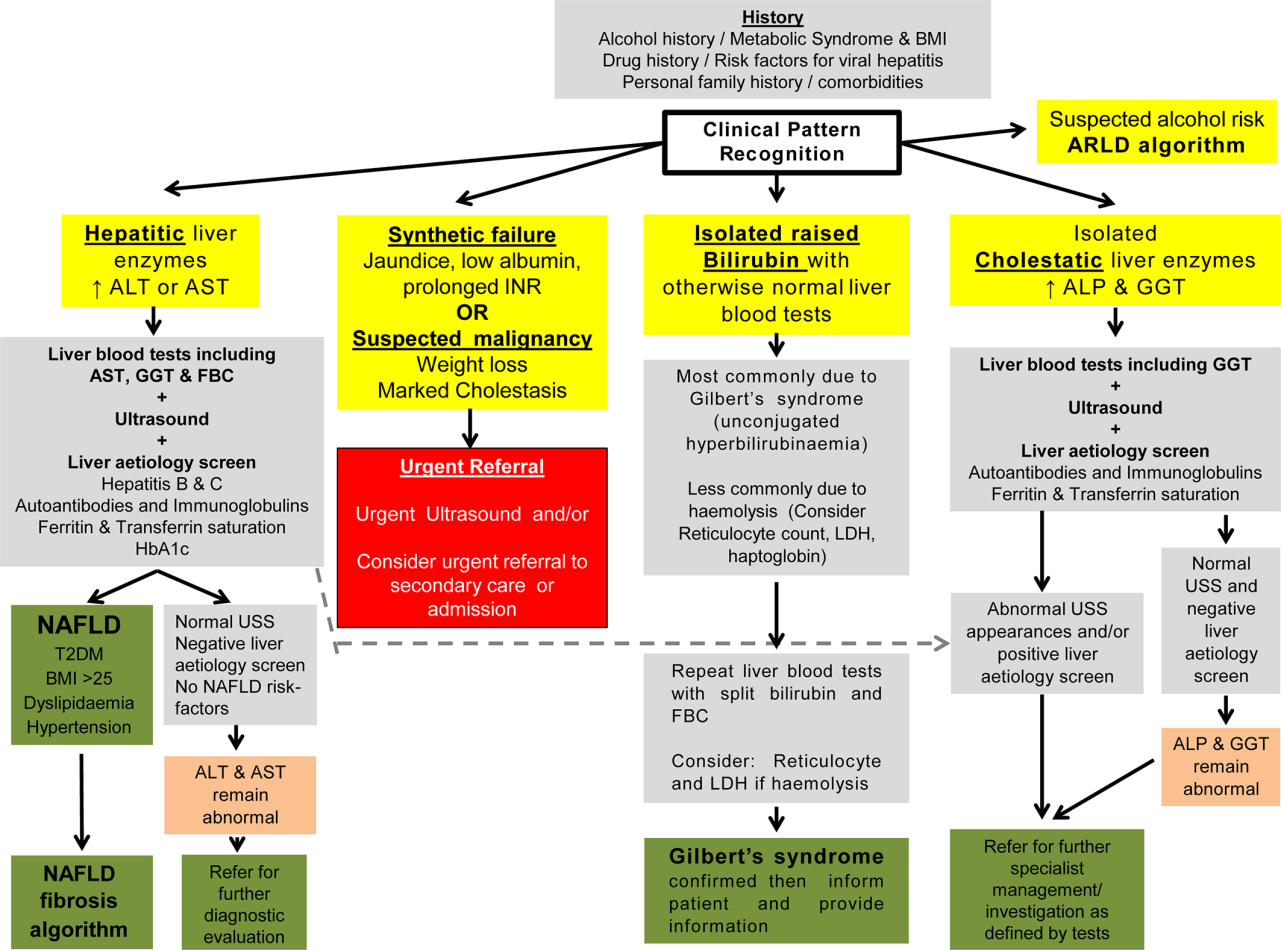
Response to abnormal liver blood tests. This figure details the initial response to abnormal liver blood tests. Boxes in yellow indicate the initial evaluation of the clinical presentation. Patients with marked derangement of liver blood tests, synthetic failure and/or suspicious clinical symptoms/signs should be considered for urgent referral to secondary care (red box). For the remainder, a clinical history alongside evaluation of the pattern of liver blood test derangement will determine choice of pathway and is shown in the grey boxes. A grey box indicates all the tests that should be requested at that stage rather than a hierarchy within it. The presence of metabolic syndrome criteria should be sought to support a diagnosis of NAFLD. For children, the text should be consulted for modification of recommendation. Areas of diagnostic uncertainty are indicated in orange boxes and the decision for repeat testing or referral to secondary care will be influenced by the magnitude of enzyme elevation and clinical context. Green boxes indicate final/definitive outcomes for users of the pathway. *Abnormal USS may well include extrahepatic biliary obstruction due to malignancy, which should result in urgent referral. ALP, alkaline phosphatase; ALT, alanine aminotransferase; ARLD, alcohol-related liver disease; AST, aspartate aminotransferase; BMI, body mass index; FBC, full blood count; GGT, γ-glutamyltransferase; INR, international normalised ratio; LDH, lactate dehydrogenase; NAFLD, non-alcoholic fatty liver disease; T2DM, type 2 diabetes mellitus; USS, ultrasound scan.
